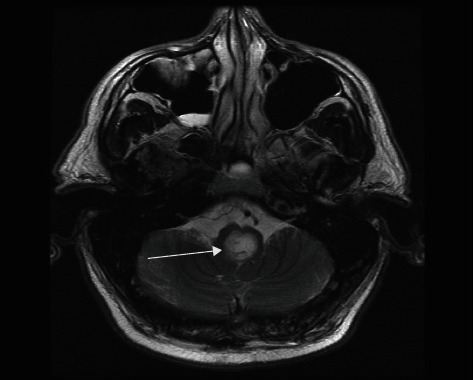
Preoperative MR brain T2-weighted axial image demonstrates a large cyst in the caudal medulla.
